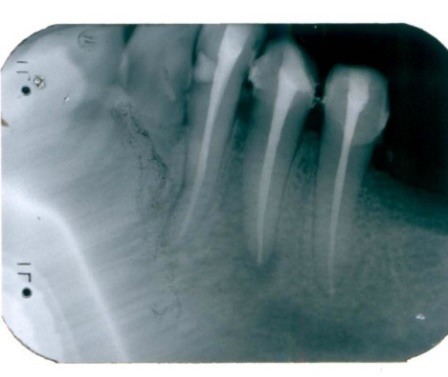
Tooth 3.4 reduced periapical radiolucency
